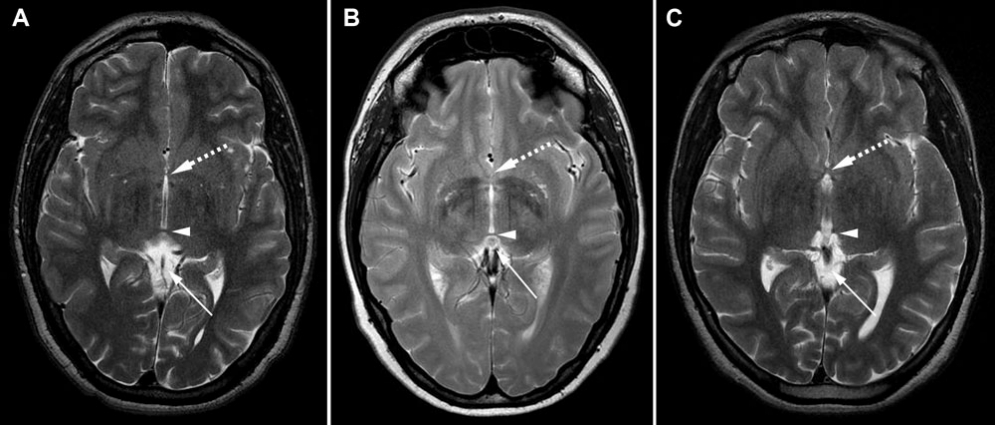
Axial cerebral T2-weighted magnetic resonance images. A: Patient III-1 from Family 1. Dashed arrow: severe hypoplasia of the anterior commissure. Arrow head: normal posterior commissure. Lower arrow: absence of the pineal gland. B: Normal magnetic resonance imaging (MRI) images. Dashed arrow: normal anterior commissure. Arrow head: normal posterior commissure. Lower arrow: normal pineal gland. C:  Patient IV-3 from Family 2. Dashed arrow: normal anterior commissure. Arrow head: absent posterior commissure. Lower arrow: absent pineal gland.
